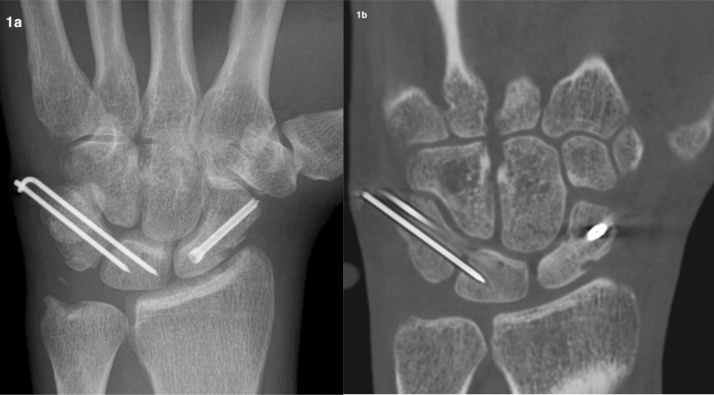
Manual worker aged 34 years with an unstable fracture in Schoenberg’s zone III of the right scaphoid according to Mayo criteria [21] treated with surgery to stabilize the fracture. XR (a) showed no healing, according to all readers. To the contrary, CBCT (b) showed total agreement of scaphoid consolidation.
